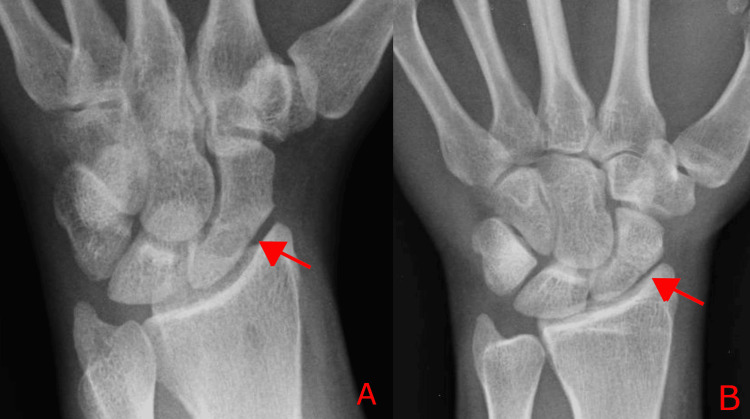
Initial wrist radiographs demonstrating a minimally displaced scaphoid fracture (A–B) Posteroanterior in ulnar deviation and posteroanterior radiographs. Arrows indicate the scaphoid fracture.
